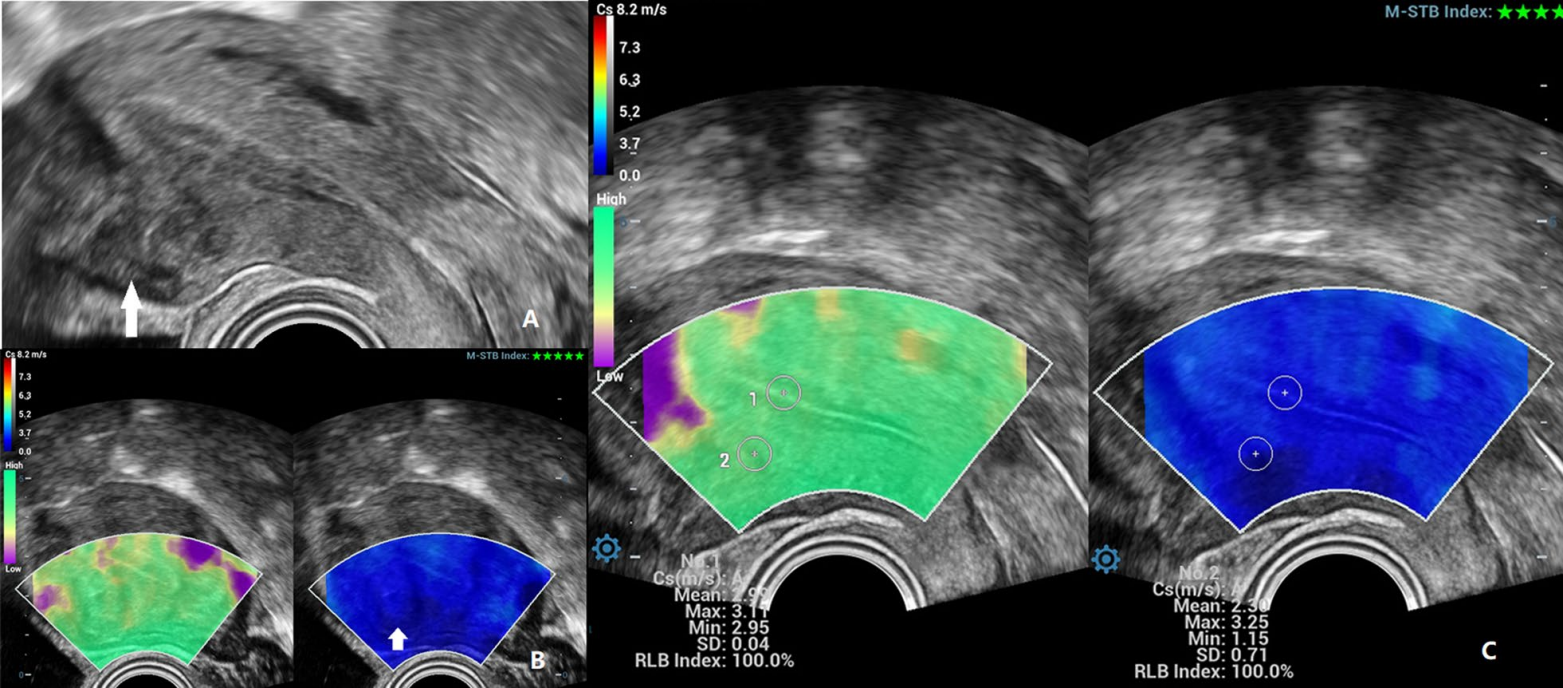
SWE used to diagnose of uterus fibroids. A Transvaginal ultrasound showed a hypoechoic lesion in the anterior inferior uterine segment (marked with a white arrow). B SWE showed a lighter blue color pseudocapsule that circling around the fibroid (marked with a white arrow). C Locating the region of interest at the lesion 2 and shear wave speed (Cs) measured automatically
